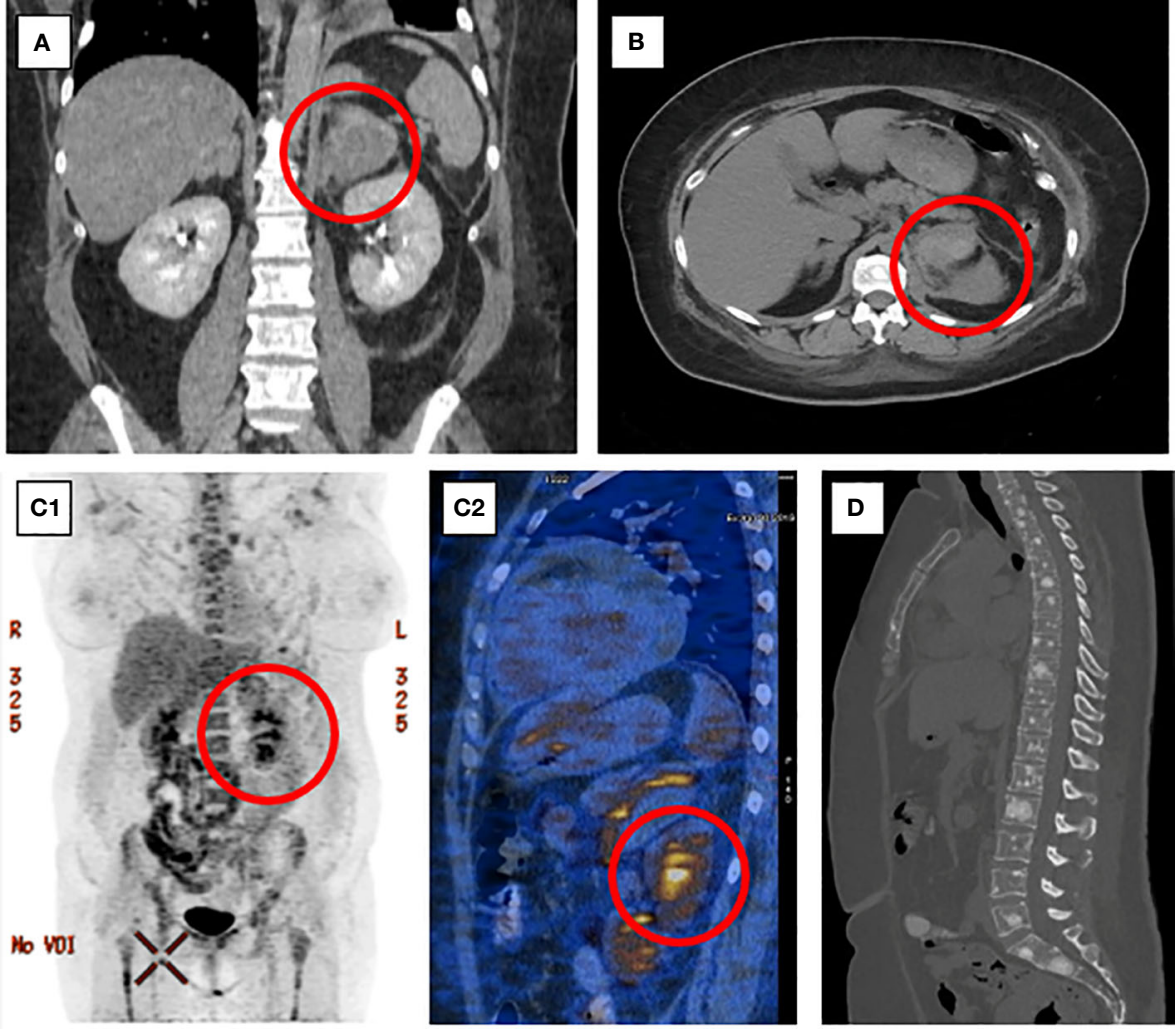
Frontal (A) and axial (B) CT scans of the abdomen presenting a large lesion of the left adrenal gland. 18F-FDG-PET-CT presenting high metabolic activity of the left adrenal gland lesion (C1, C2) and metastatic bone lesions in the spine and sternum (D).
